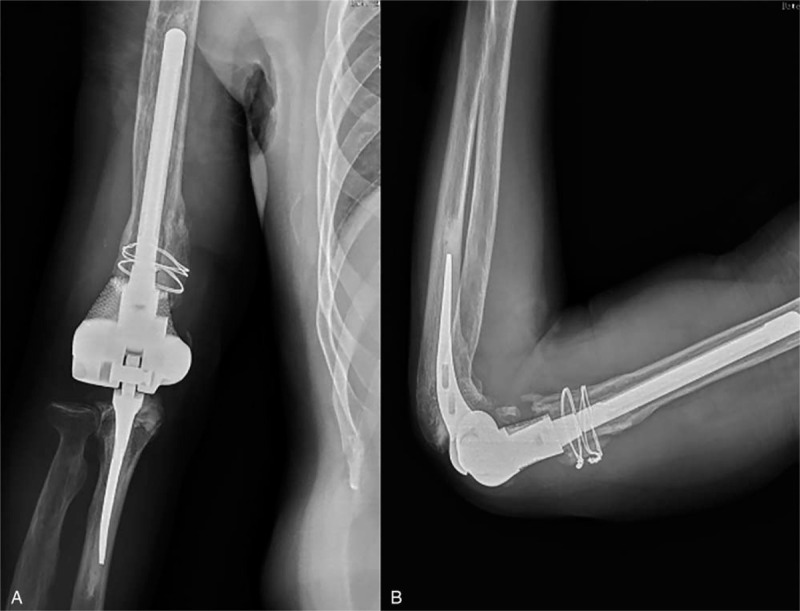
Plain radiographs in the 36 months follow-up. (A) Anteroposterior plain radiograph of the right elbow joint. (B) Lateral plain radiographs of the right elbow joint.
